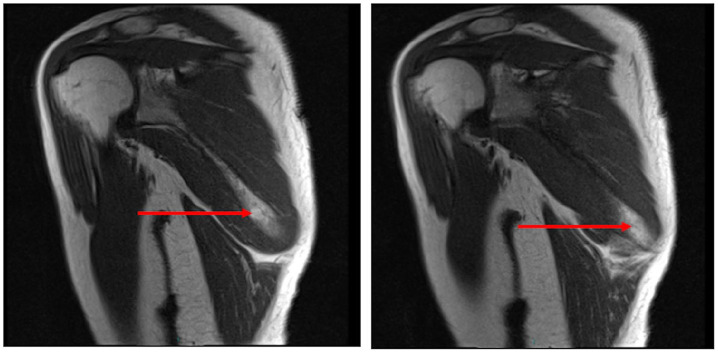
T1-weighted MRI images of right scapula. Standard sequences without contrast sagittal T1-weighted images of right scapula. A focal bone marrow oedema of approximately 2 cm is highlighted at the inferior margin and the inferior pole of the right scapula (red arrows). In addition, there is slight oedema of the adjacent muscles around the inferior scapular margin in keeping with enthesopathy/tendinopathy.
